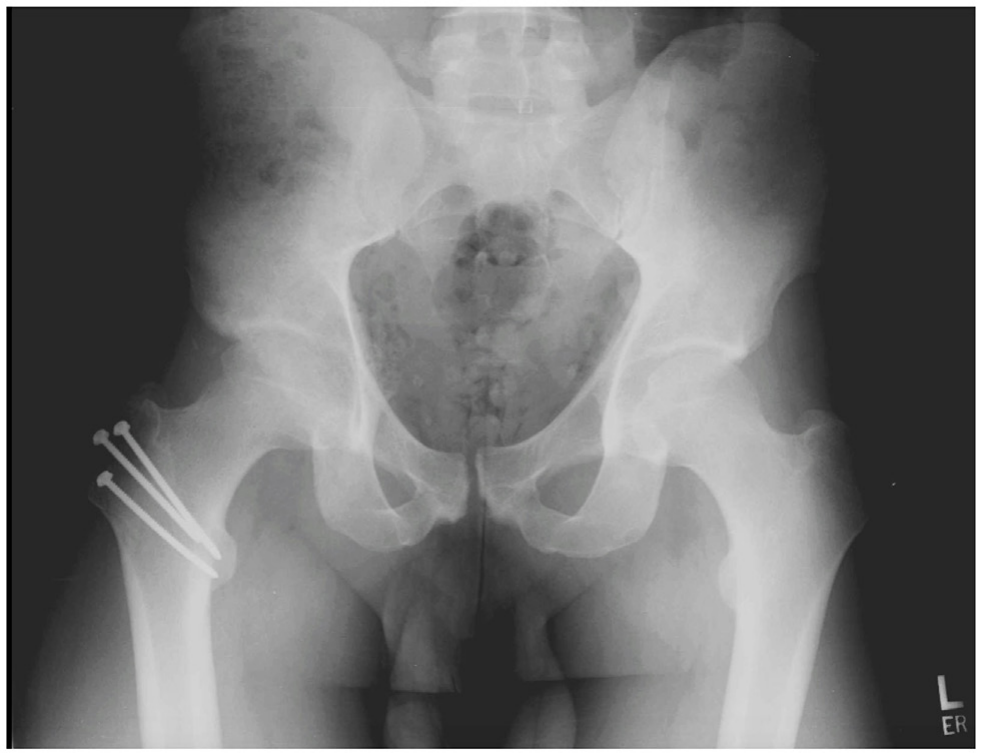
A postoperative radiograph of a surgical hip dislocation secured with screws.
